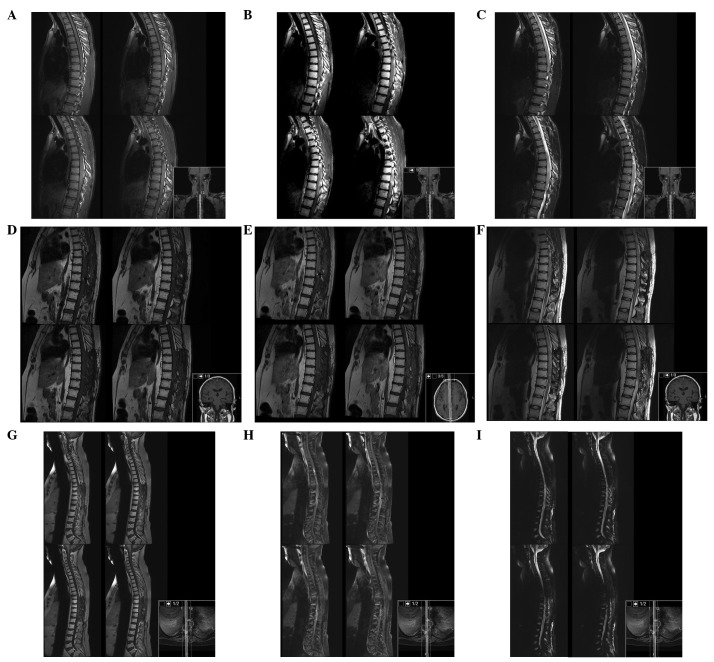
Spine MRI. (A) T1, (B) T1 with contrast and (C) T2-weighted dorsal spine sagittal view MRI at presentation, demonstrating the presence of the tumour between T1 and L1. (D) T1, (E) T1 with contrast and (F) T2-weighted dorsal spine sagittal view MRI 3 months after surgery. (G) T1, (H) T1 with contrast and (I) T2-weighted dorsal spine sagittal view MRI 17 months after surgery, demonstrating enlargement of the enhancing mass from T3 to T12 with subarachnoid metastatic deposit in C2 and C4. MRI, magnetic resonance imaging.
